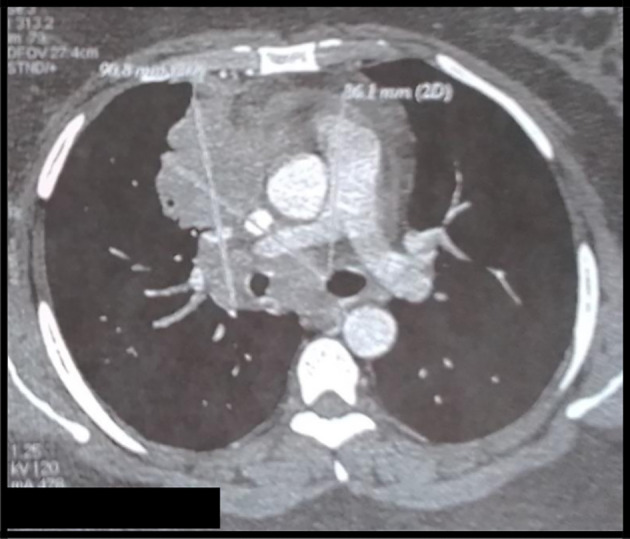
CT mediastinal section showing an important mediastino-hilar mass infiltrating the right main bronchus.
